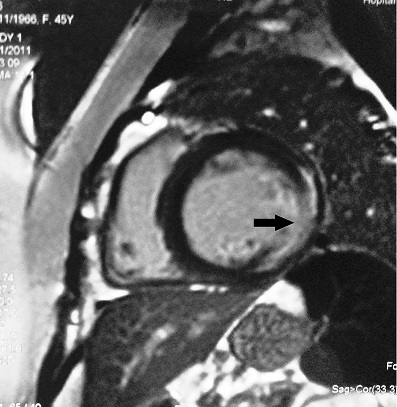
Decreased uptake in the inferolateral and apical ventricular myocardium, which was reversible during stress (reverse distribution) shown on Technetium99m sestamibiscintigraphy myocardial perfusion study
